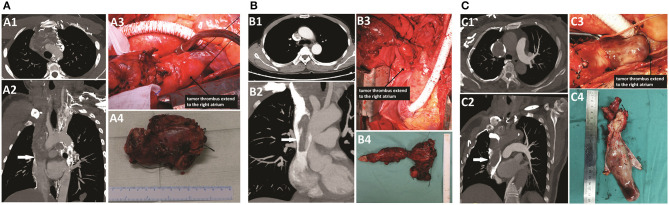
CTA scan and intraoperative findings of patients. (A) Patient 1: A1–A3, an extended tumor thrombus totally occupied the left brachiocephalic vein and the SVC, near the right atrium (white arrow). A4, the resected vessels invaded by the tumor thrombus. (B) Patient 2: B1–B3, an extended tumor thrombus occupied the left brachiocephalic vein and the SVC, near the right atrium, but the obstruction of the SVC segment was incomplete (white arrow). B4, the resected vessels invaded by the tumor thrombus. (C) Patient 3: C1–C3, an extended tumor thrombus totally occupied the right brachiocephalic vein and the SVC, near to the right atrium (white arrow). C4, the resected vessels invaded by the tumor thrombus.
